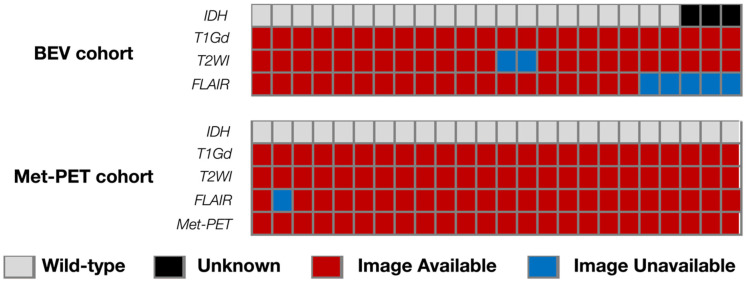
Overview of the analyzed cohort with the landscape of genetic information and availabilities of MRI sequences. The study prepared two cohorts: the BEV cohort with nCET after BEV administration and the Met-PET cohort with Met-PET.
